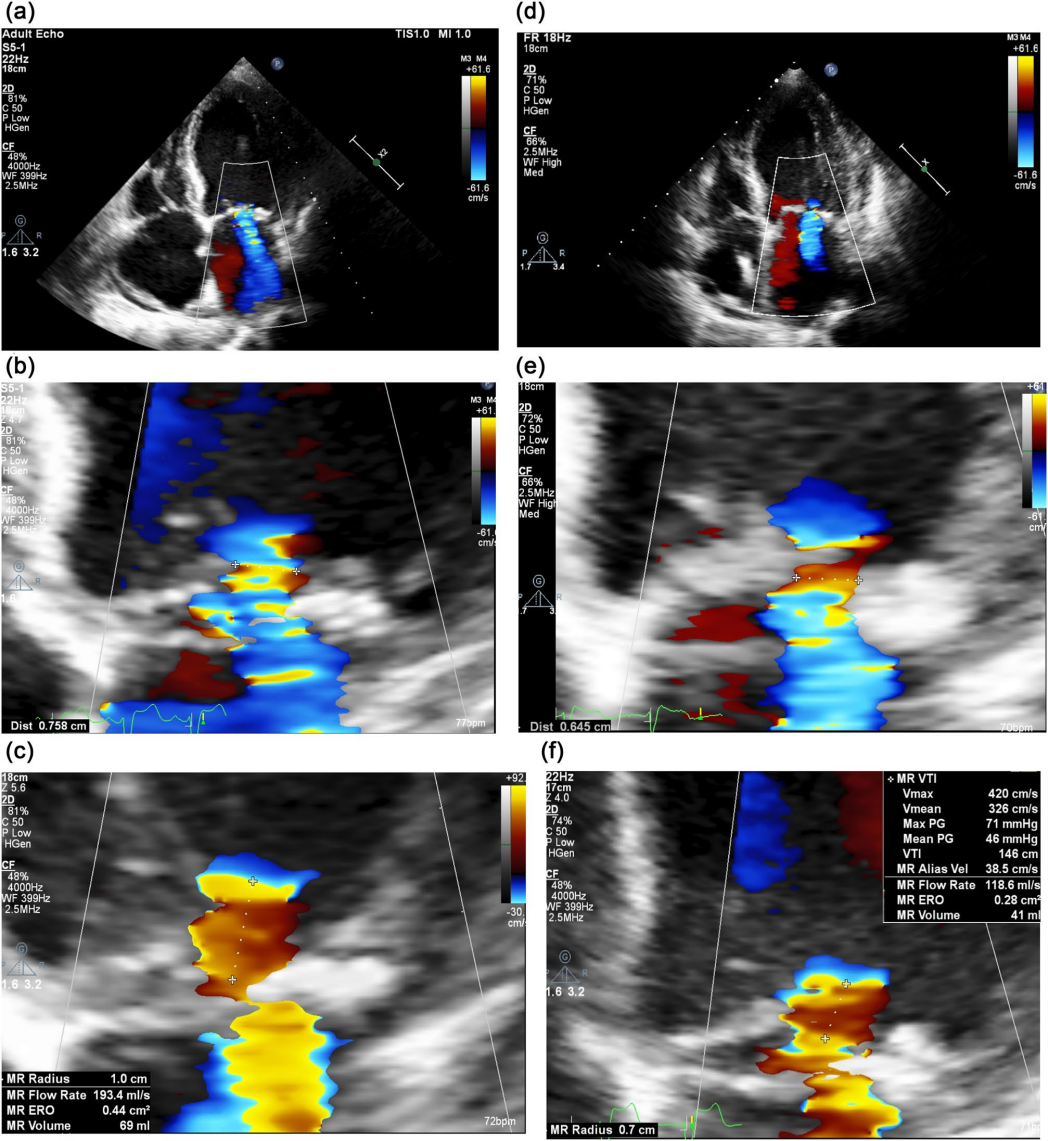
Images showing changes in the severity of mitral regurgitation during the treatment of ADHF. Initially severe mitral regurgitation—a, b and c in echocardiographic assessment on discharge was evaluated as moderate—(d), (e) and (f)
